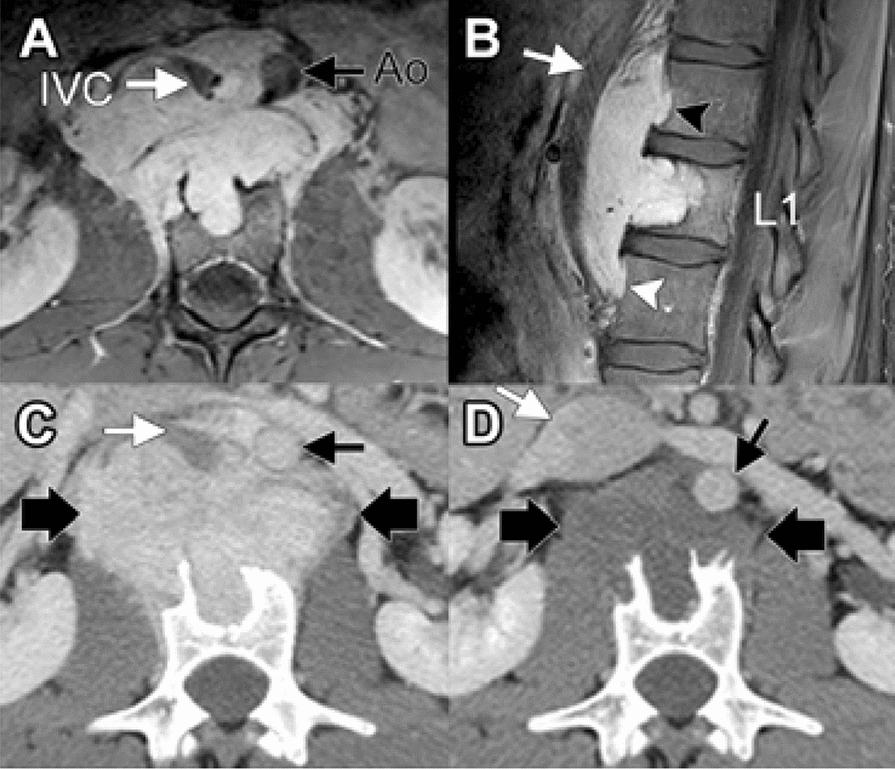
Imaging features of the lesion. a, b Axial and sagittal post-contrast MRI demonstrates an avidly enhancing lesion in the L1 pre-vertebral soft tissues. There is anterior displacement of the aorta (Ao, black arrow) and inferior vena cava (IVC, white arrows). There is invasion of the L1 vertebral body, with cephalad and caudad extension of disease and secondary pressure erosion of the ventral cortices of T12 (black arrowhead) and L2 (white arrowhead) vertebral bodies. c Concurrent contrast-enhanced CT image at the level of L1 shows an avidly enhancing mass (between large black arrows) with invasion of the L1 vertebral body. The CT shows rim of sclerosis in the vertebral body indicative of secondary invasion from a soft tissue mass. The aorta and inferior vena cava (black and white arrows, respectively) are anteriorly displaced. d Contrast-enhanced CT at end of therapy shows significant decrease in size and degree of enhancement of the mass (between large black arrows). Mass effect on the aorta and inferior vena cava (black and white arrows, respectively) has also decreased
